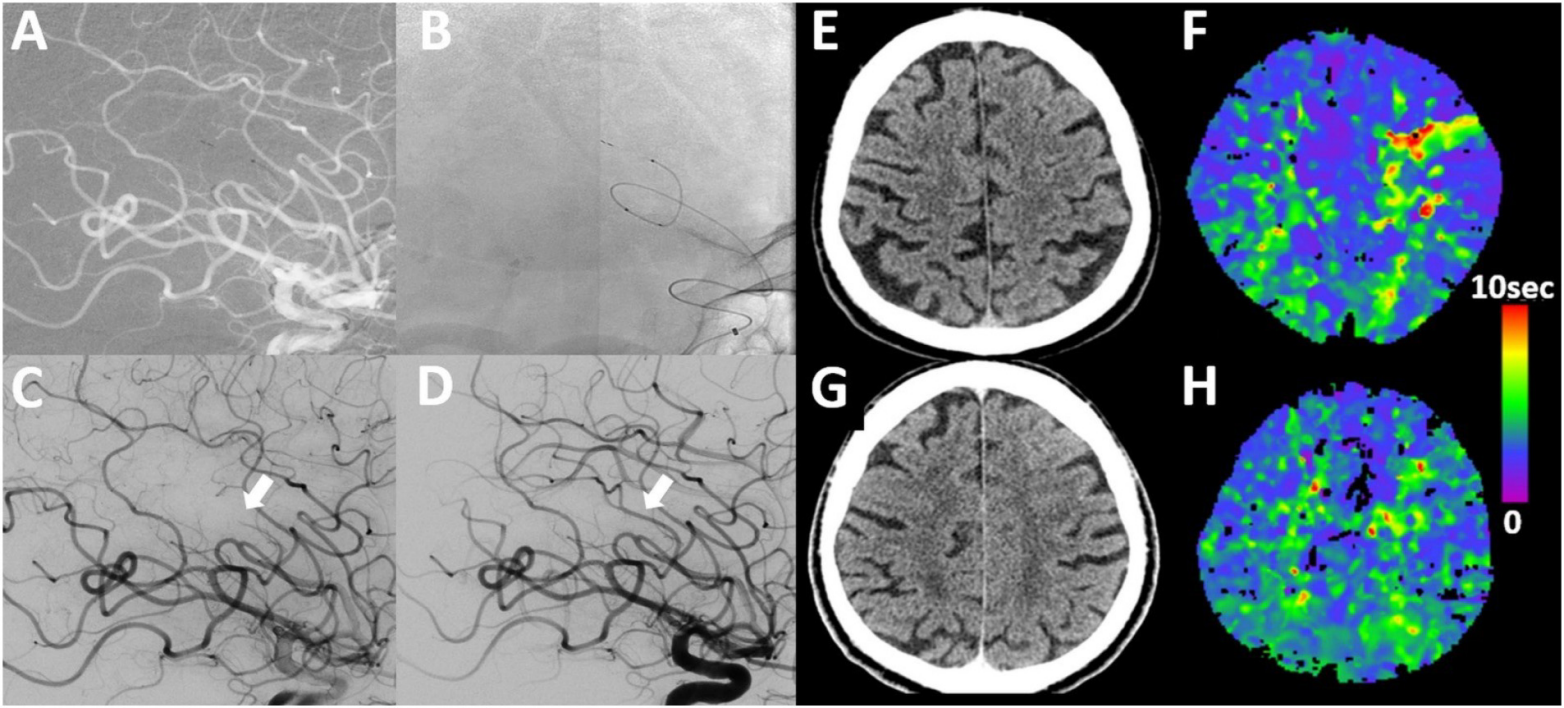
Example of SPORNS technique in a patient with M4 occlusion. A male patient presenting with NIHSS 5 (right-sided weakness and incomplete aphasia). (A) Lateral DSA of distal M4 occlusion which was not seen on CT-angiography but CT-perfusion without early infarct lesion in CT (E, F (TMax-map)). (B, C) Thrombectomy employing the SPORNS technique with partial release of the stent-retriever (pRESET lite 3–20 deployed through SL 10 microcatheter), resulting in complete recanalization (D). On 24 h follow-up, there are no signs of infarct or hemorrhage in non-contrast CT and CT-perfusion shows no signs of previous cortical ischemia in the left hemisphere (G, H).
